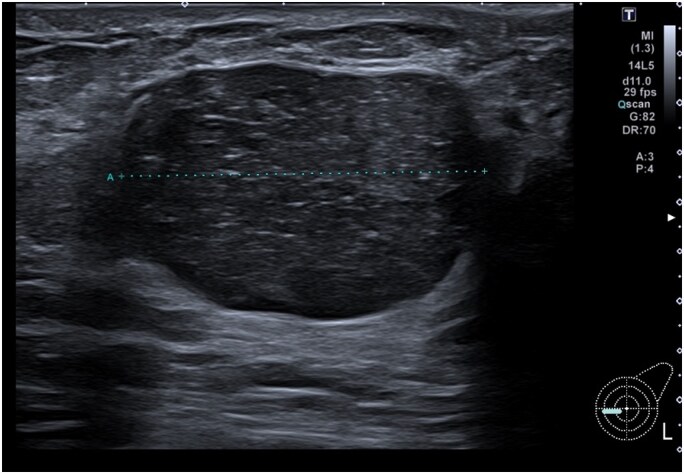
Ultrasound images of an upgraded lesion (B3-FA to intermediate Phyllodes Tumor). Neither size nor ultrasound score was predictive of upgrade.
